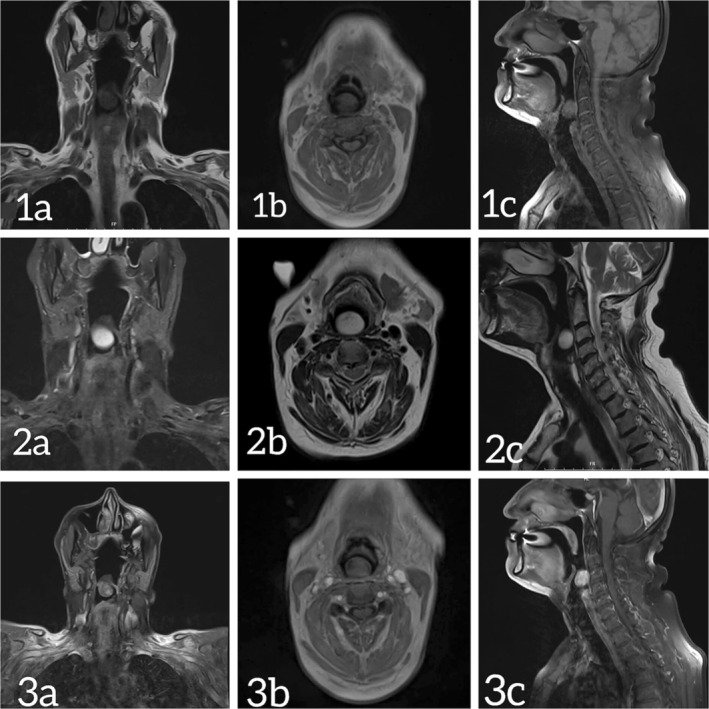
MRI of the neck demonstrates a well‐circumscribed, heterogeneous lesion in the posterior hypopharynx. Images 1, 2, and 3 represent the T1‐weighted, T2‐weighted, and gadolinium‐enhanced T1‐weighted sequences, respectively. Panels a, b, and c show the coronal, axial, and sagittal planes, respectively.
